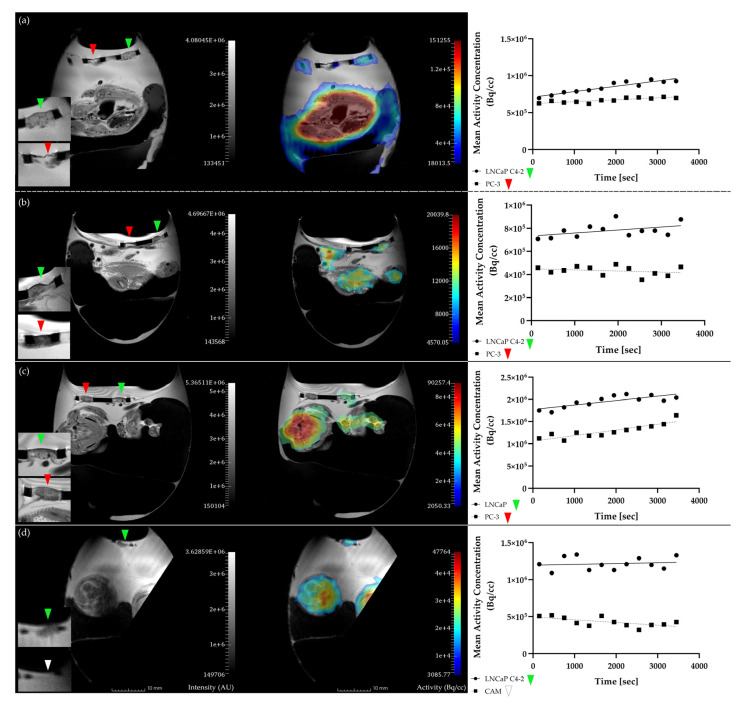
Magnetic resonance (MR) images (T2-weighted, TSE [28], left panel) and PET/MR fusion images (middle panel) of hen’s egg test–chorioallantoic membrane (HET-CAM) xenograft experiments with the corresponding time–activity curves for the respective PET region of interest (right panel). The PSMA-positive tumors can be clearly visualized in the MR image in all cases ((a,b,d): LNCaP C4-2, (c): LNCaP), whereas the negative tumor PC-3 can only be seen in examples (a–c) and did not grow in example d (PSMA^+^ → green arrow; PSMA^−^ → red arrow; CAM → white arrow). Note the more intense accumulation in the PSMA-positive tumors as compared to the PSMA-negative tumors, suggesting target-specific tracer accumulation.
